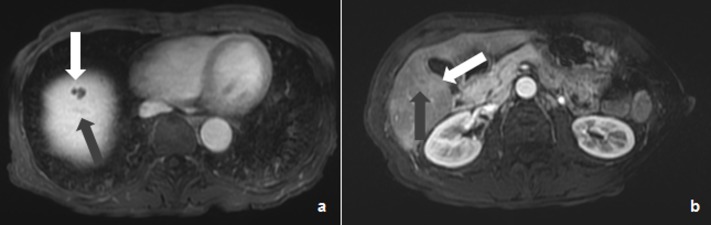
Post-chemotherapy LAVA MR imaging (August 2017) Cystic lesion (white arrow) and residual VIII segment lesion (grey arrow) (a) and cystic lesion (white arrow) and residual V segment lesion (grey arrow) (b). MR: magnetic resonance
